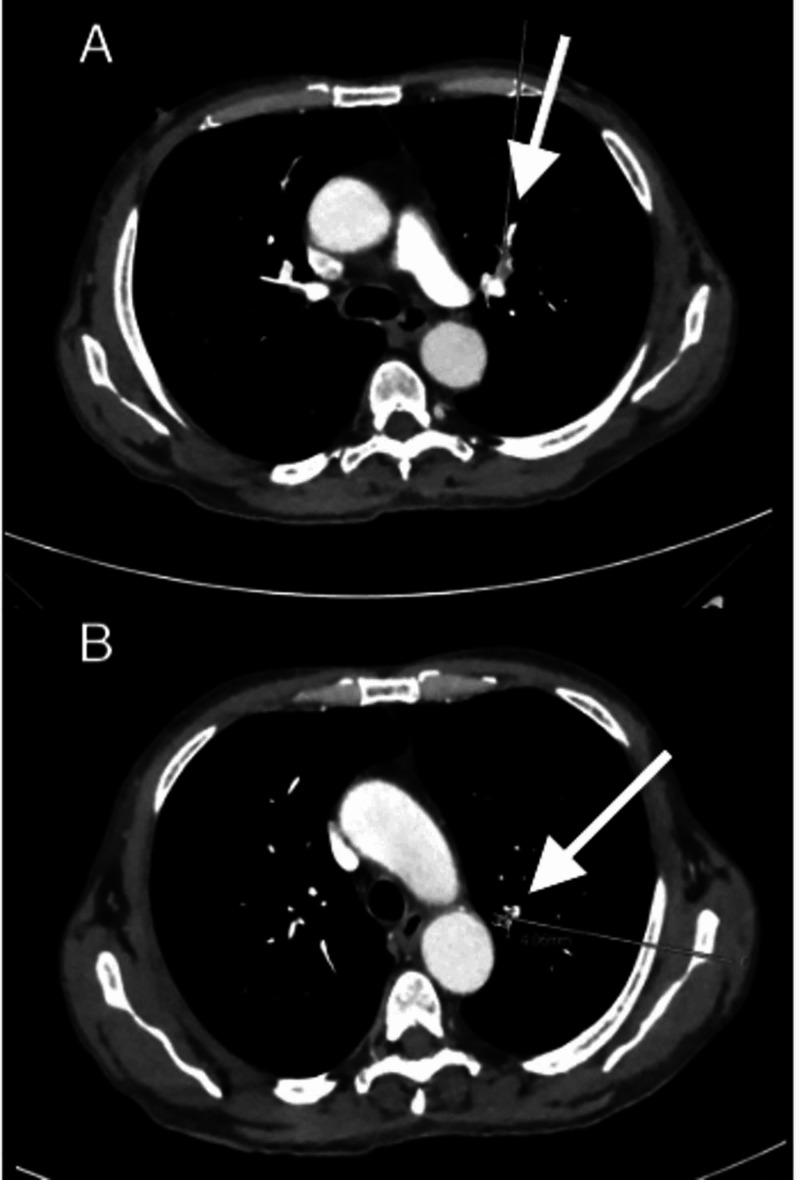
Chest CT scan with contrast. A: subsegmental pulmonary embolus (arrow). B: indeterminate pulmonary nodule (arrow).
